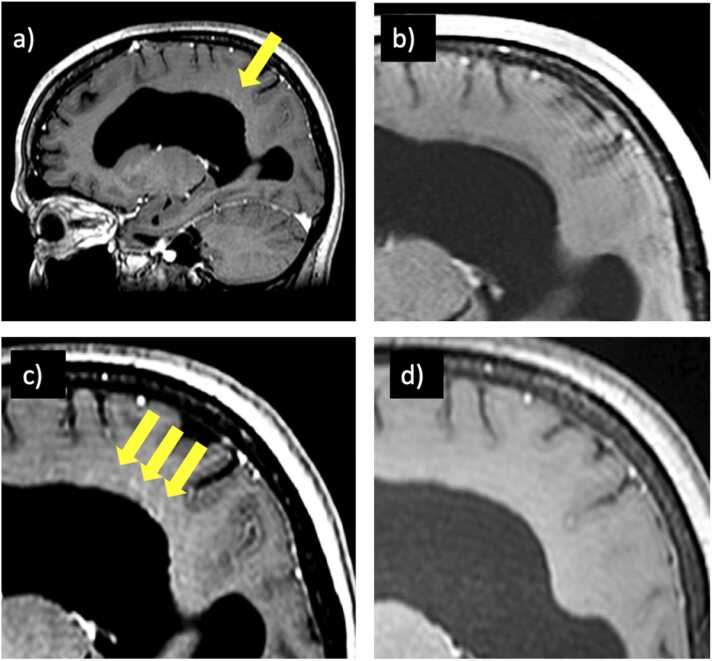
A patient with anti-GFAP autoimmune encephalitis. (a) Whole-brain sagittal contrast-enhanced T1 image demonstrating linear strands of contrast enhancement radiating outwards from the ventricle. This finding was not seen on initial MRI (b). (c) Cropped, zoomed image of (a) performed on day 95 where the finding was first noted. (d) Resolution of findings upon second-line treatment with rituximab.
